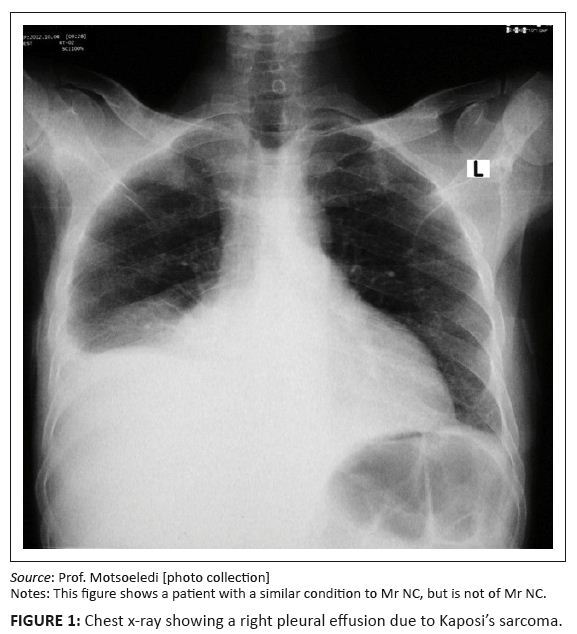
Chest x-ray showing a right pleural effusion due to Kaposi’s sarcoma. *Source:* Prof. Motsoeledi [photo collection] Notes: This figure shows a patient with a similar condition to Mr. NC, but is
